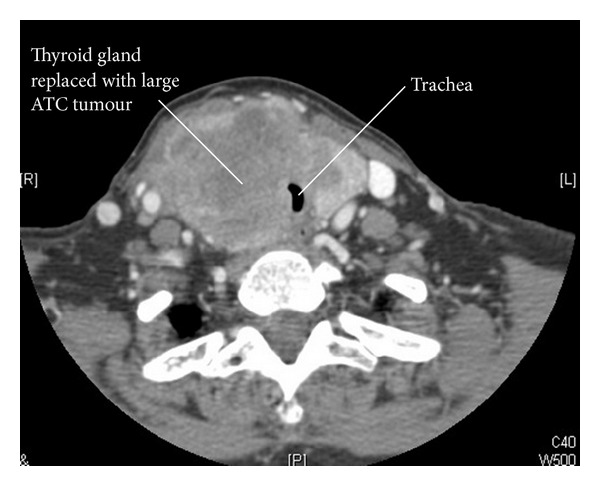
CT scan of patient with ATC causing tracheal compression.
